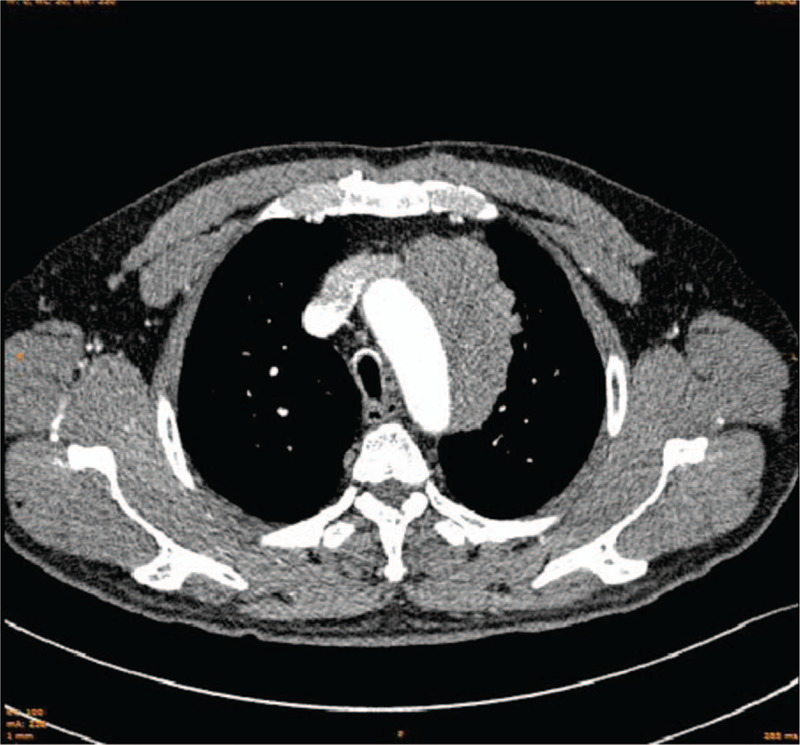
Enhanced CT showed malignant tumor in the middle and upper mediastinum on the left side.
